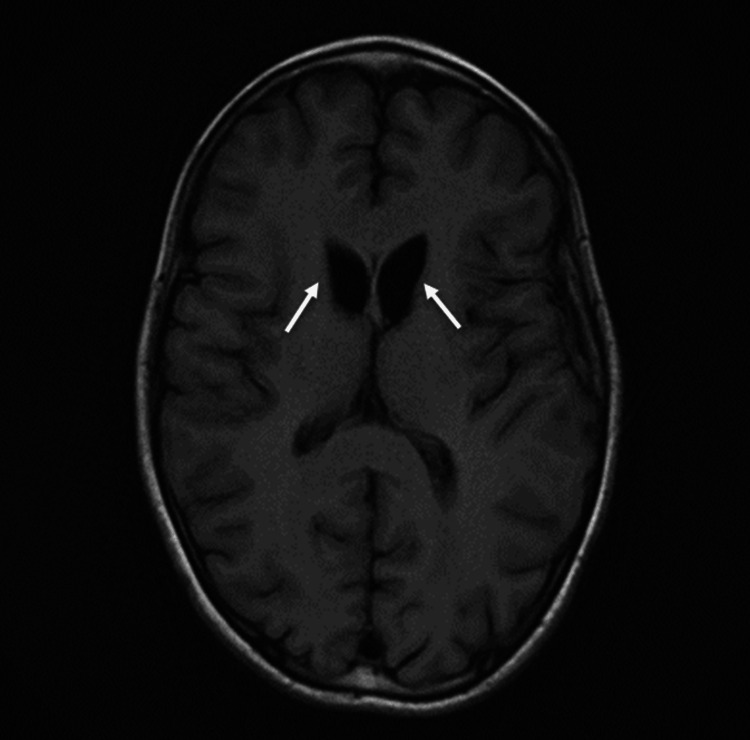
Axial T1-weighted MRI of the brain shows volume loss in the bilateral putamen and caudate heads (white arrows).
